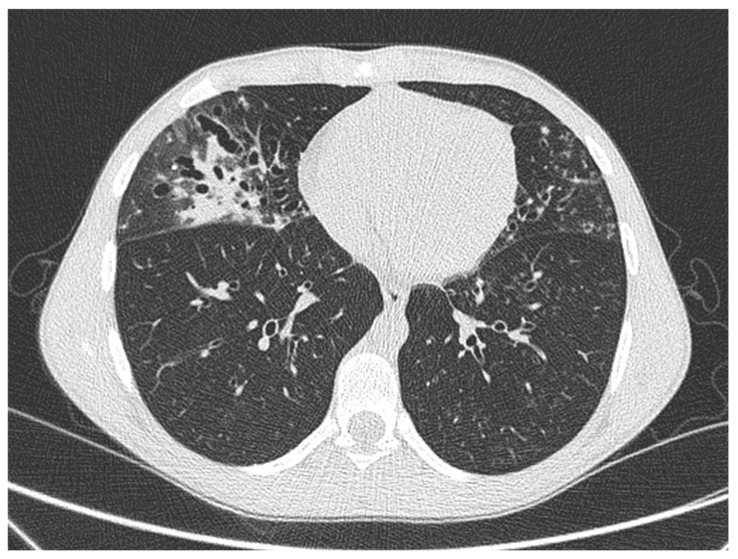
Chest CT in a male young adult affected by PCD, showing bronchiectasis in the middle lobe, with concomitant consolidation and mucous plugs, and new “tree-in-bud” opacities in the lingula.
